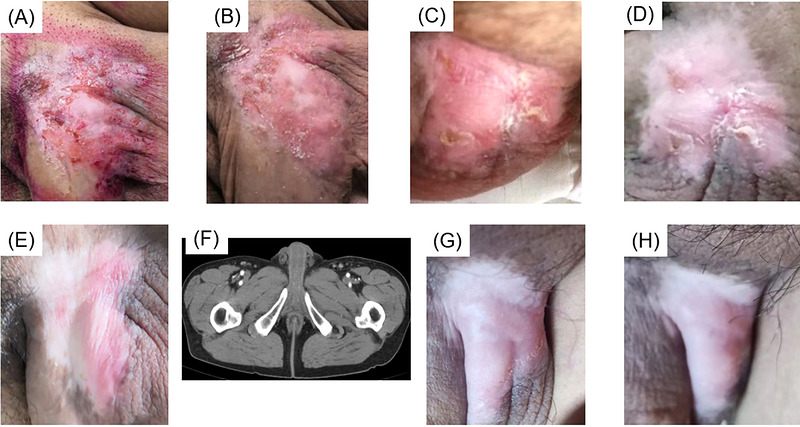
The grade of acute radiation‐induced adverse effects and tumor control after the administration of FLASH‐RT. (A) 1‐week post irradiation; (B) 3‐weeks post irradiation; (C) 6‐weeks post‐irradiation; (D) 2‐months post‐irradiation; (E) 3‐months post‐irradiation; (F) CT images of 3‐months post‐irradiation; (G) 6‐months post‐irradiation; (H) 12‐months post‐irradiation. (Fig. 3A, 3B, and 3E were previously published in Journal of Zhengzhou University (Medical Sciences), and reprinted permission was approved).
